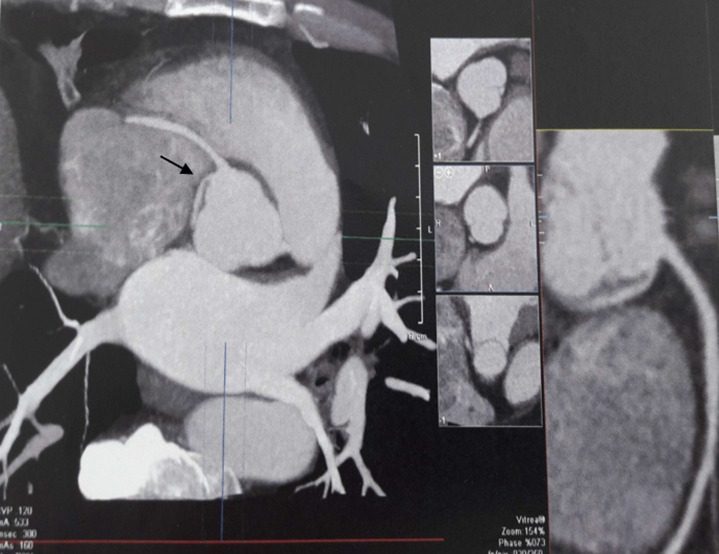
patient #8 CT coronary angiography revealing the connexion of the circumflex artery to the right sinus with a proximal path between the aorta and the right atrium (arrow) without an intramural segment
